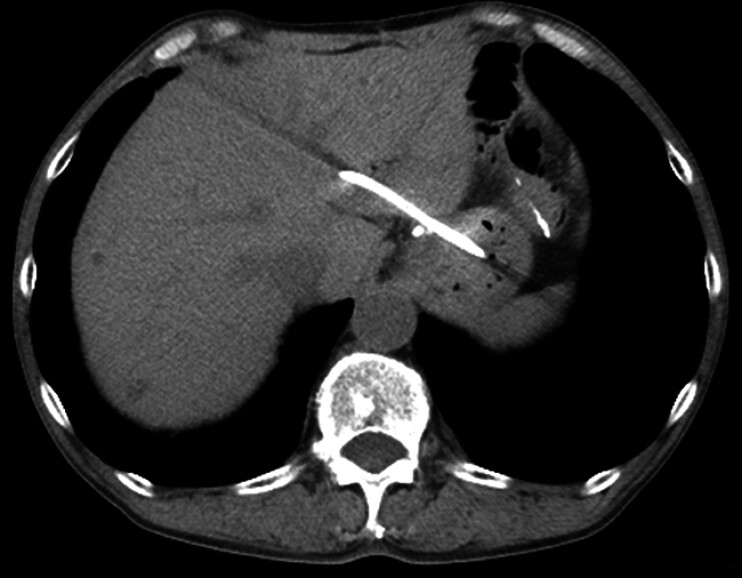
Abdominal computed tomography 1 day after endoscopic ultrasound-guided hepaticogastrostomy showing a plastic stent placed from the stomach to the intrahepatic bile duct.
